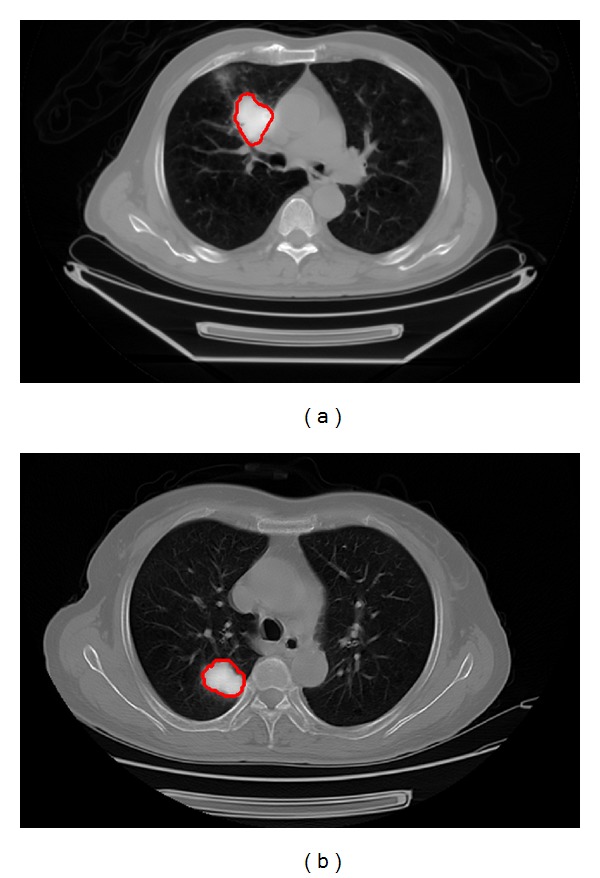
Fused PET/CT images of two patients with lung tumors delineated by radiation oncologist shown in red lines.
